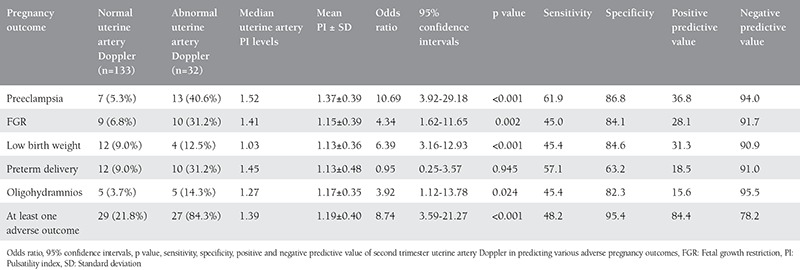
Normal and abnormal second-trimester uterine artery Doppler in adverse pregnancy outcomes and median uterine artery Doppler pulsatility index value
